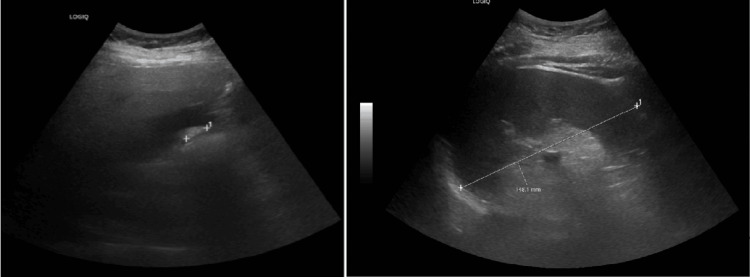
Ultrasound abdomen showing echogenic sludge noted within the gallbladder lumen (left) and enlarged spleen (right).
